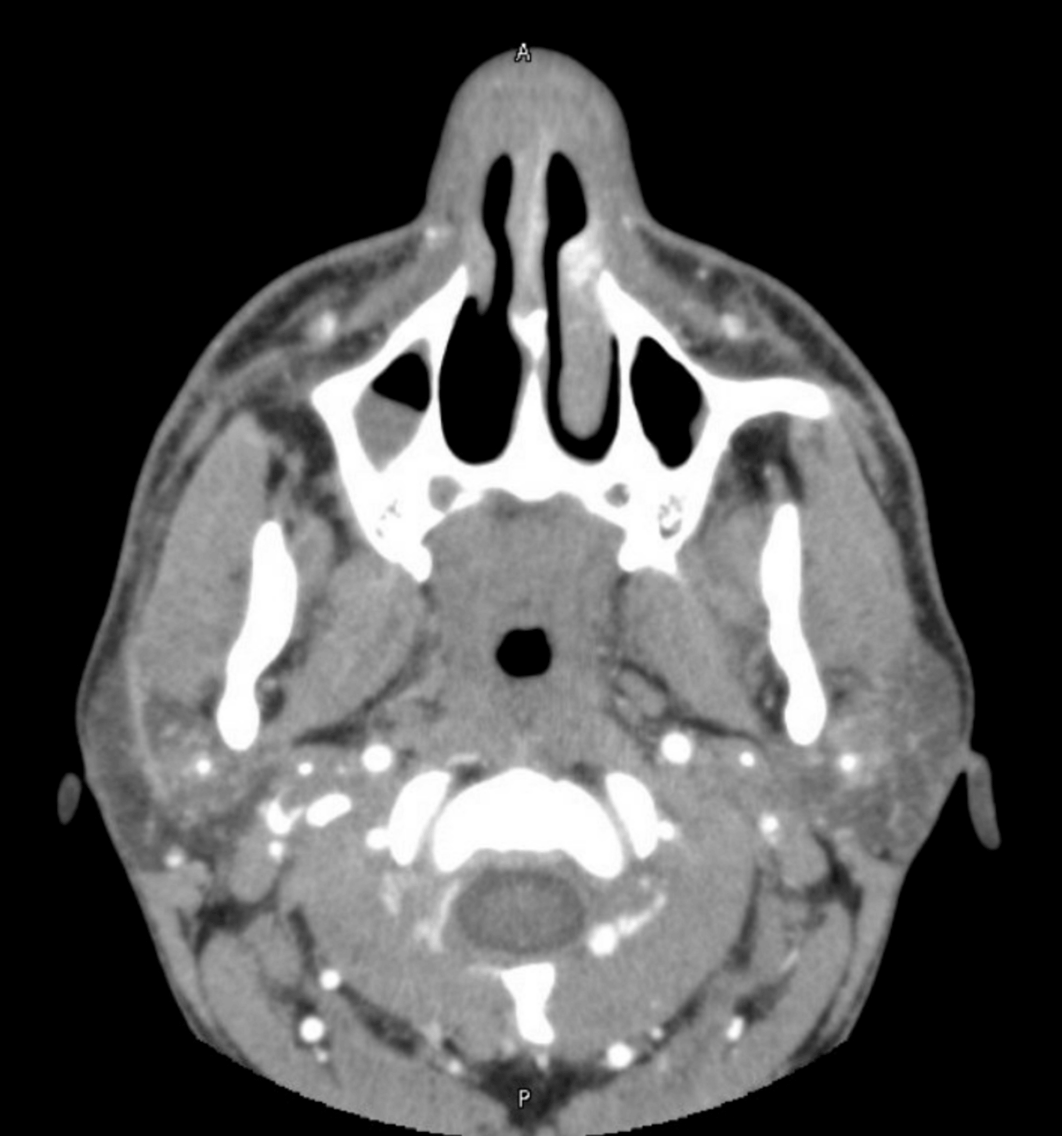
CT scan showing NSA NSA, nasal septal abscess
